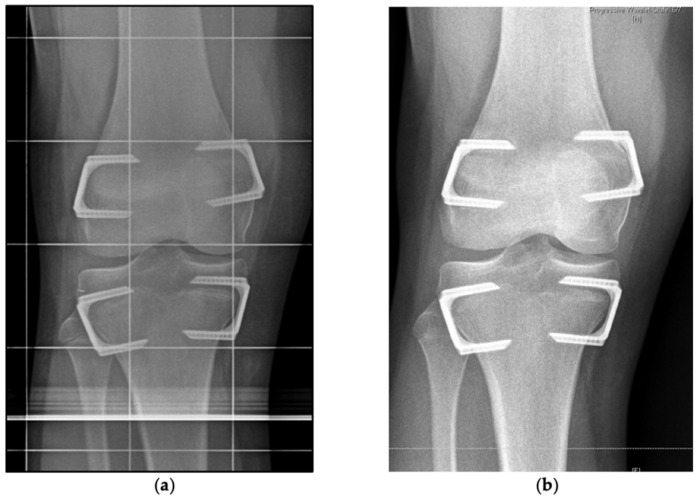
Proximal tibial epiphysiodesis without proximal fibular epiphysiodesis. (a) After implantation: Anteroposterior radiograph showing proximal tibial epiphysiodesis without proximal fibular epiphysiodesis on a right knee performed with RigidTacks^TM^ in a 14-year-old boy with LLD of 2.3 cm. (b) Before implant removal: Same patient after a treatment time of 19.6 month with proximalization of the fibular head right before implant removal. The fibular head is localized higher than the center of the proximal tibial growth plate (dPTFH = 1.9 mm).
